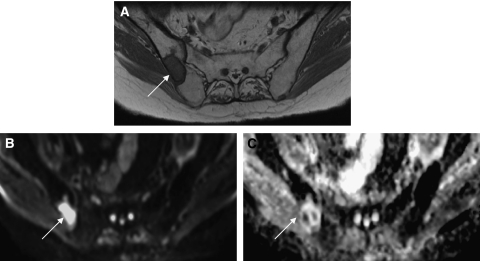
A male patient with prostate cancer metastases to bone. T1W axial MRI pelvis (A) shows a metastasis within the right iliac bone (arrow). High signal within the lesion on the diffusion-weighted MRI of the pelvis (B) indicates that diffusion within the metastasis is less restricted than diffusion in the surrounding normal marrow. An apparent diffusion coefficient (ADC) map of pelvis (C) generated from the diffusion-weighted imaging data (B values 0, 50, 100, 250 500, and 750) provides a quantitative index of water diffusion within the tumour. The ADC map also shows heterogeneity of water diffusion within the tumour not shown by conventional T1W imaging.
